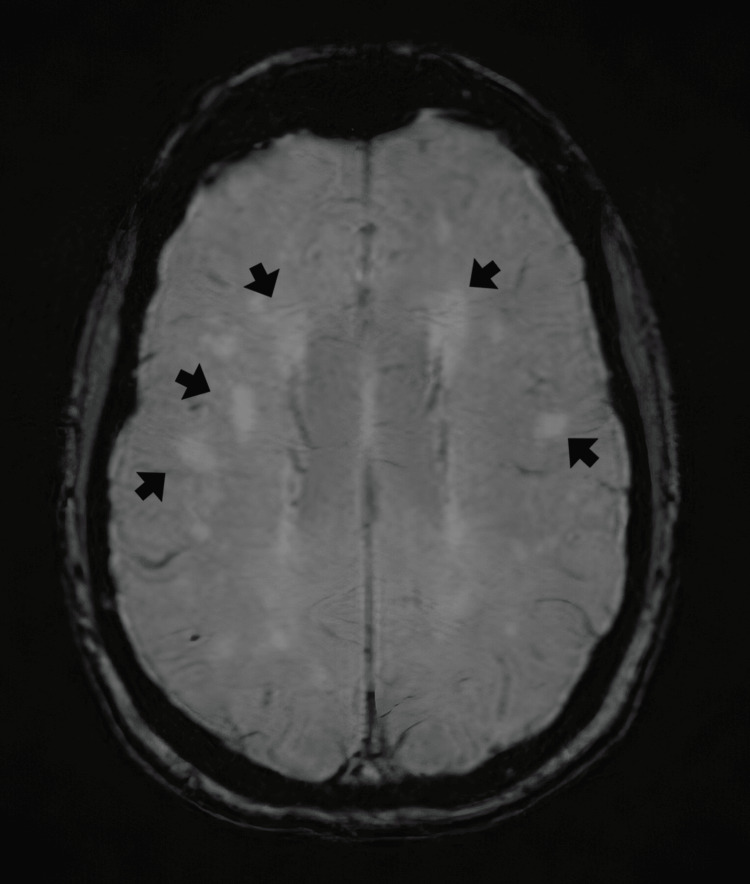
Axial T2-weighted FLAIR brain MRI image Brain MRI revealed several punctate hyperintense areas bilaterally, distributed in the frontoparietal subcortical white matter (black arrows), as well as in a periventricular distribution. These findings were interpreted as microangiopathic changes secondary to vasculitis. FLAIR: fluid-attenuated inversion recovery
